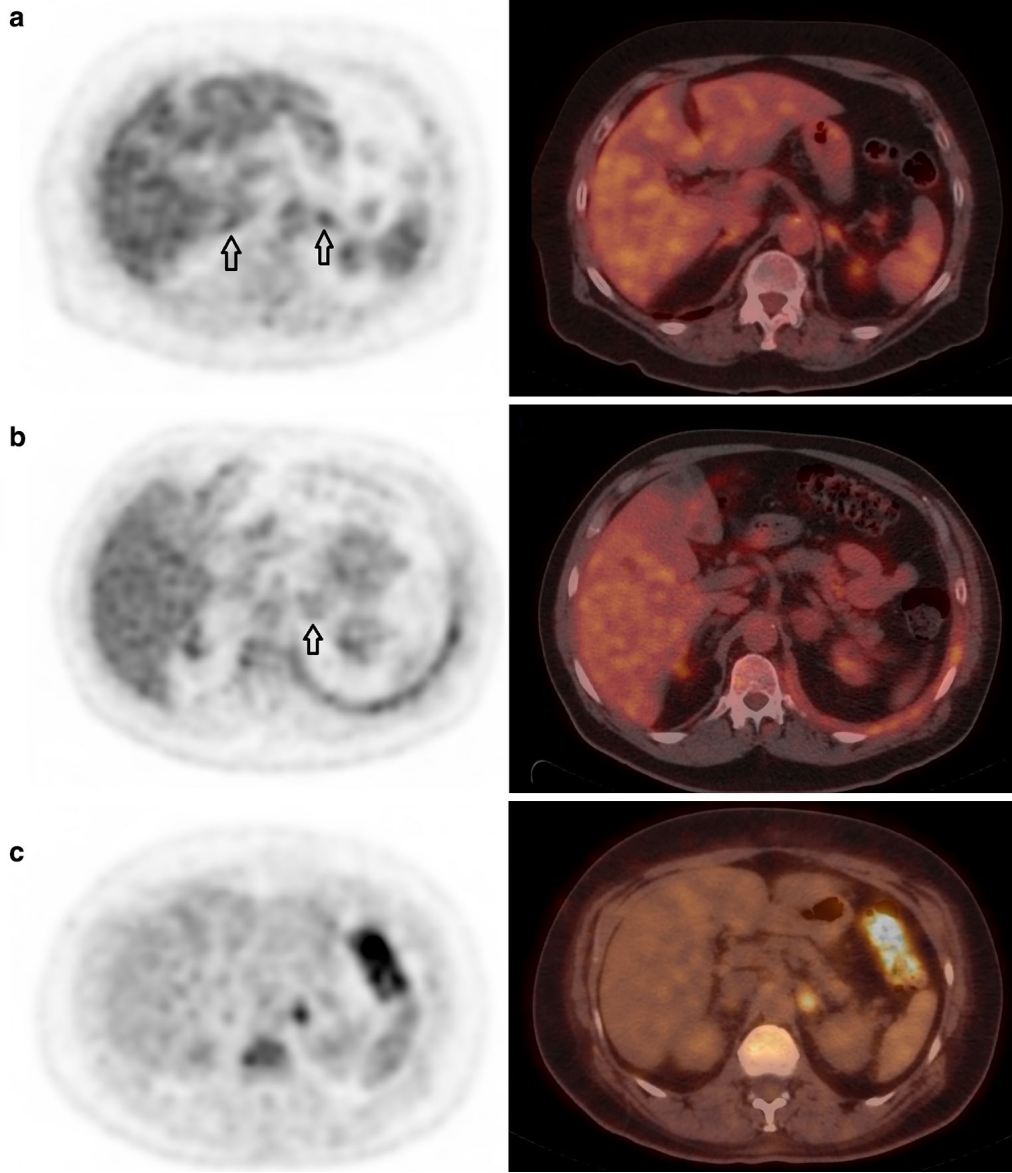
Single ^18^F-FDG PET (left) and fused ^18^F-FDG PET/CT (*right*) images of three patients. a Two normal-sized adrenal glands (*arrows*) are visualised. Uptake values for the left adrenal gland were 3.4 (SUV_max_) and 1.2 (AL ratio). For the right adrenal gland, the SUV_max_ and AL ratio were 3.2 and 1.1 respectively. A 6-months follow-up ^18^F-FDG PET/CT scan did not reveal any changes in adrenal gland size, shape and ^18^F-FDG uptake for both adrenal glands. b Patient with an enlarged left adrenal gland (*arrow*), axial diameter 32 mm. Average uptake values were 2.6 (SUV_max_) and 1.0 (AL ratio). A biopsy performed during endoscopic ultrasound was negative for tumor cells. Furthermore, 3-month follow-up ^18^F-FDG PET/CT imaging did not reveal metastatic disease in this enlarged adrenal gland. c Patient with a metastatic lesion in the left adrenal gland. On ^18^F-FDG PET images, we measured a SUV_max_ of 4.9 and an AL ratio of 3.3 for this adrenal gland. A biopsy performed during endoscopic ultrasound-guided fine-needle aspiration (EUS-FNA) confirmed the presence of an adrenal metastasis, originating from a lung tumor
